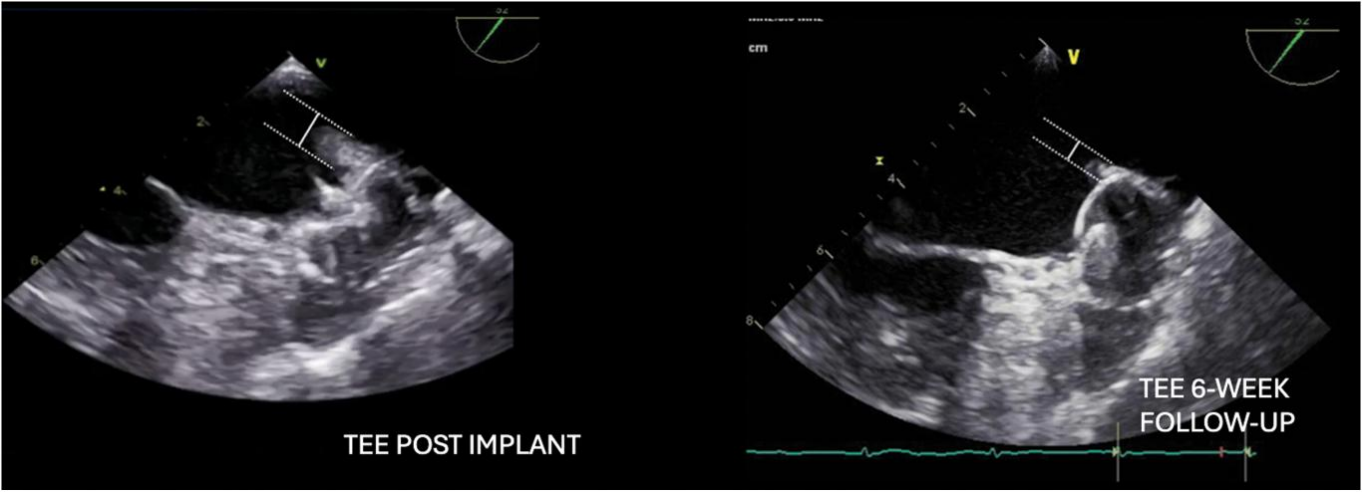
Transesophageal echo images of the marked oedema (0.8 cm) of the LAA-PV ridge observed during the procedure compared with the 6-week follow-up transesophageal echo images where the swelling completely resolved with an oedema of the measure of 0.4 cm. TEE, transesophageal echo.
